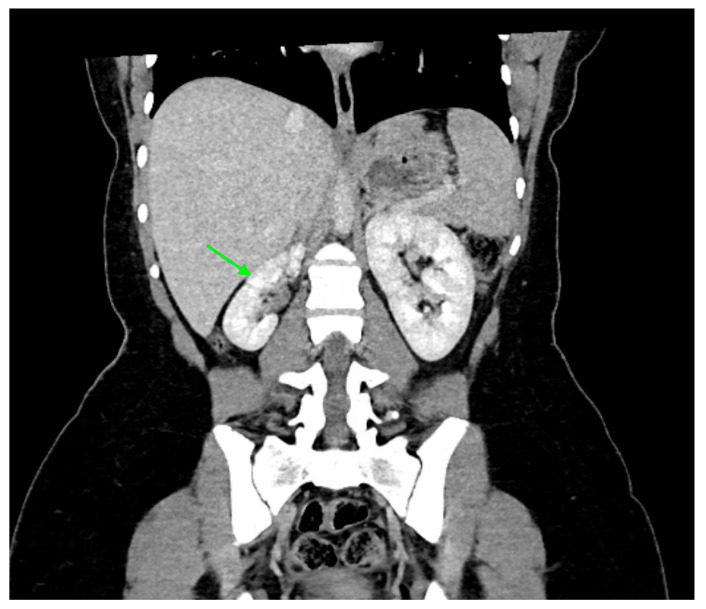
A CT scan of a 15-year-old female with a history of recurrent pyelonephritis shows a scarred and atrophic right kidney (indicated by green arrow).
